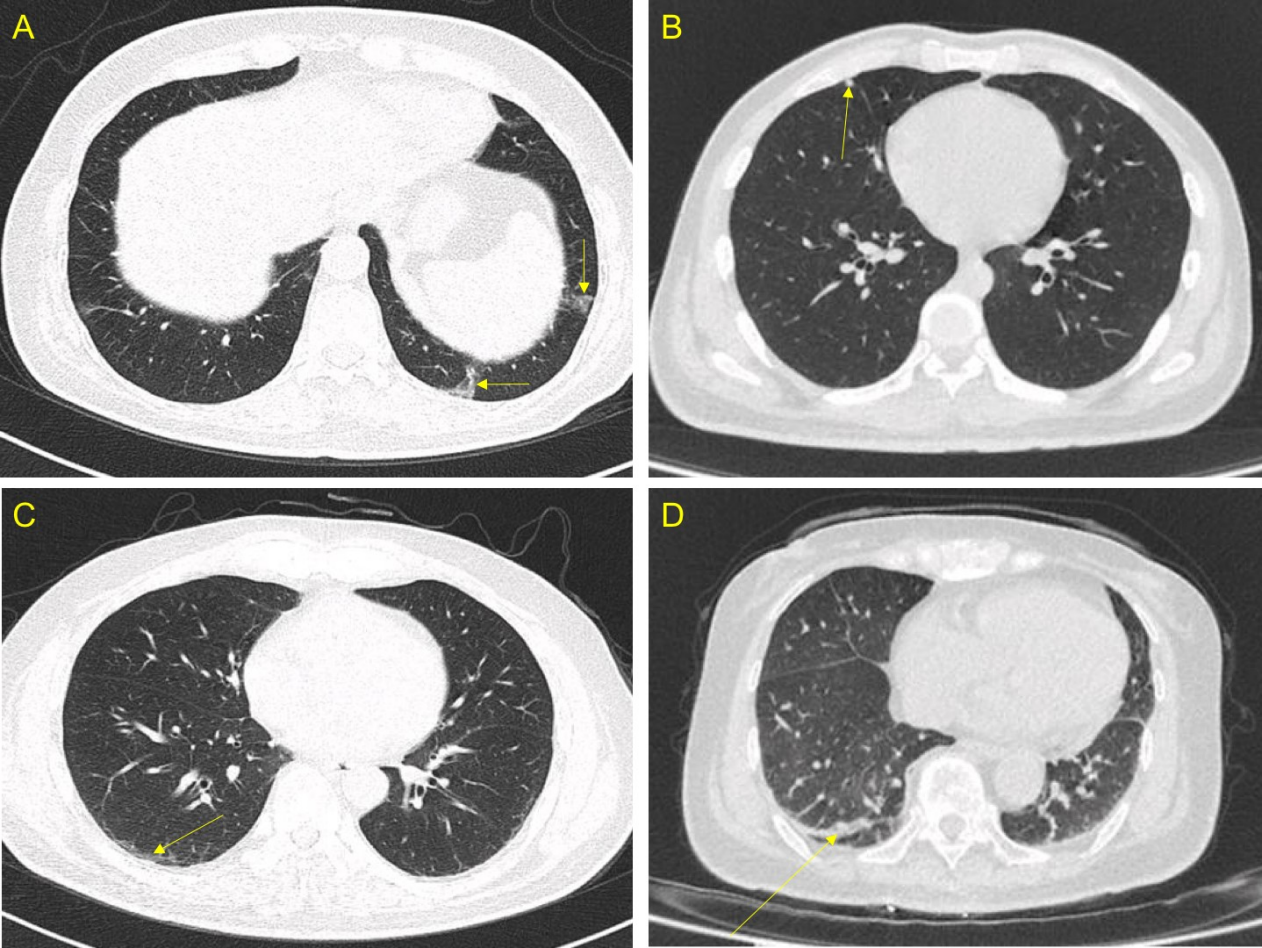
High-resolution computed tomography images. A: Ground-glass opacity (arrow); B: Solitary nodule (arrow); C: Stripe (arrow), and D: Patchy shadows and stripe (arrow).
